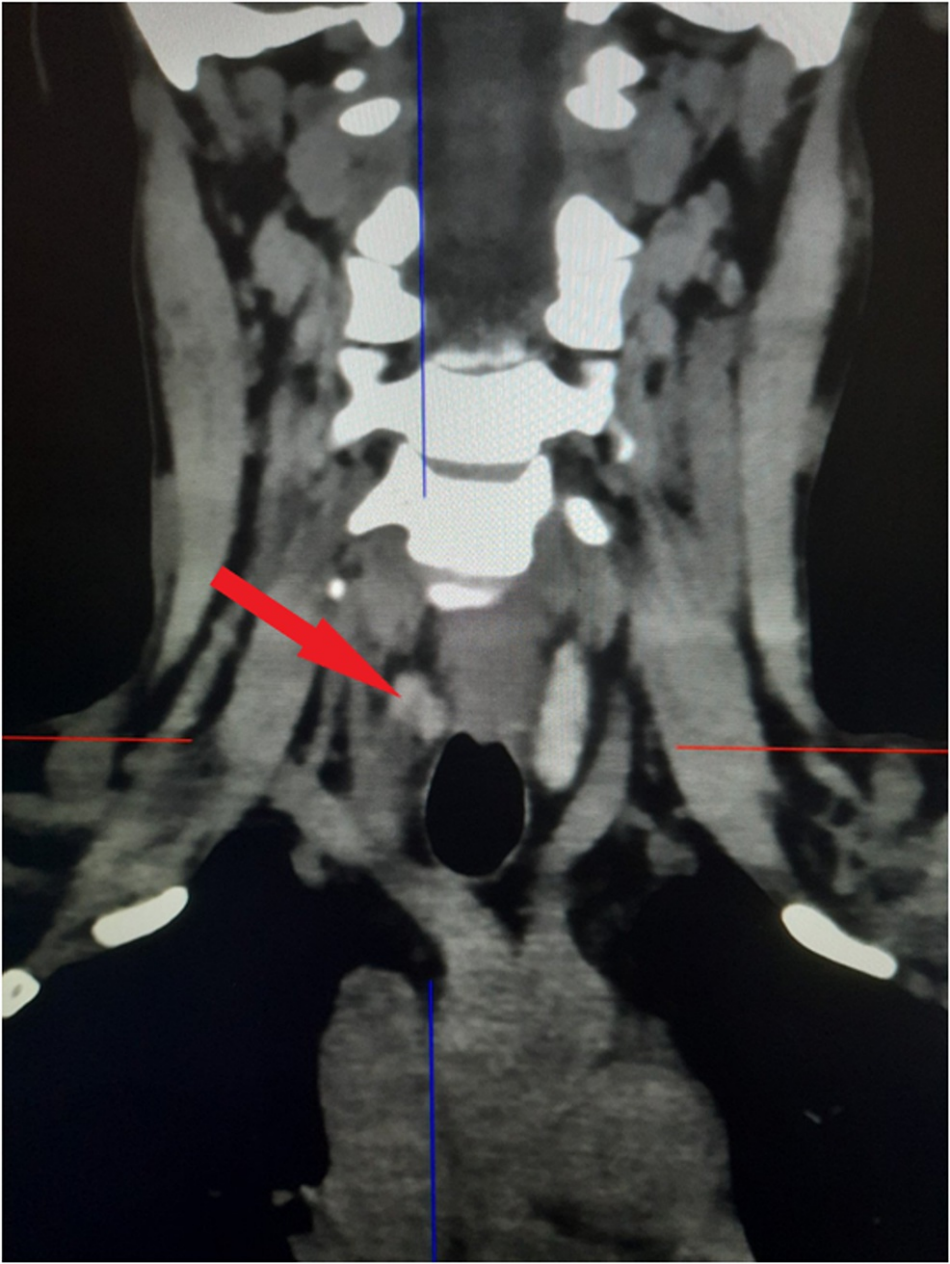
Four-dimensional computed tomography showing parathyroid adenoma (red arrow).
